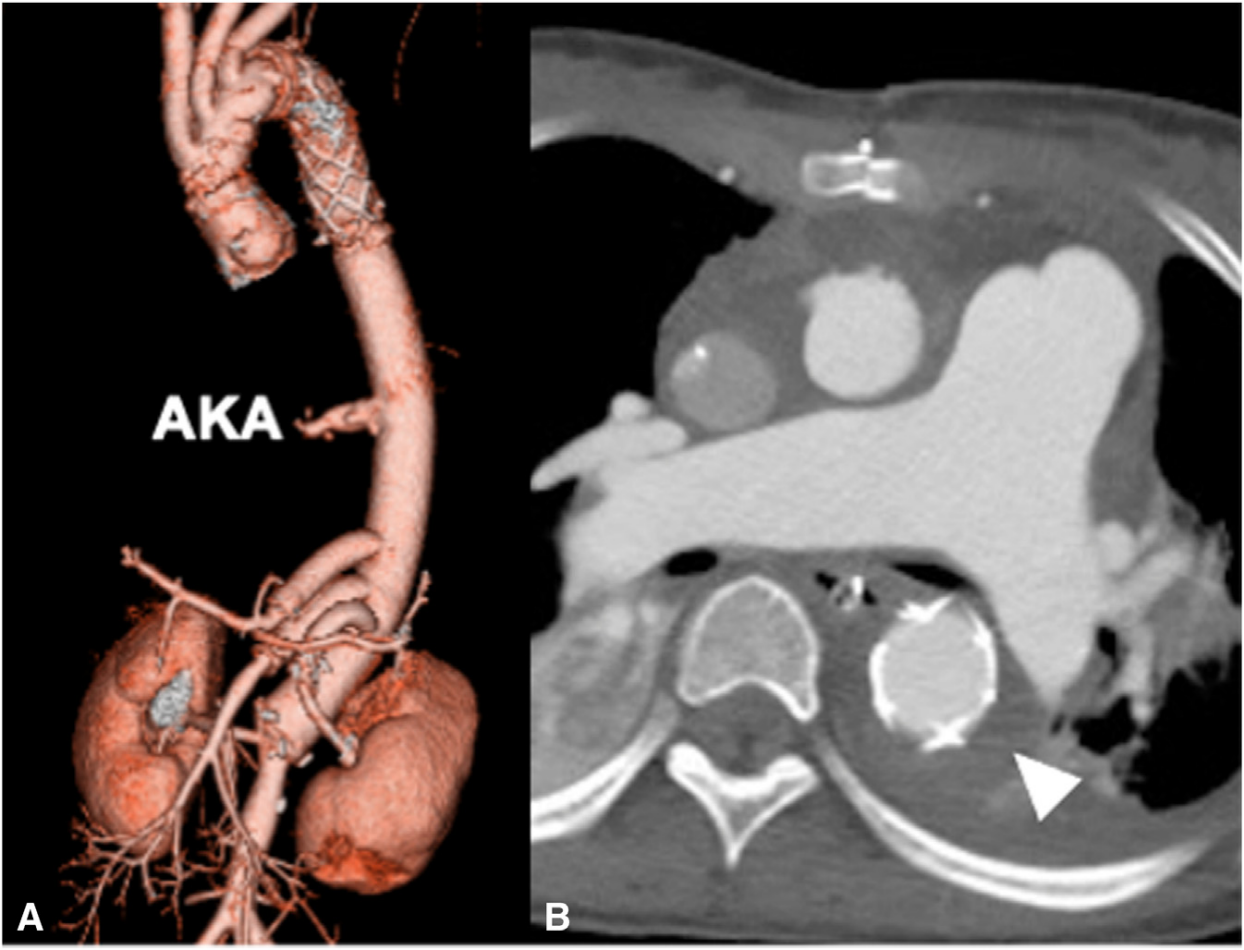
Postoperative computed tomography angiography. A, Successful staged extensive aortic replacement. Total arch replacement was performed using the frozen elephant trunk technique, which was anastomosed to a thoracoabdominal graft at the Th6 level. The Adamkiewicz artery (*AKA*) and reconstructed visceral branches were all open. B, False lumen around the frozen elephant trunk was thrombosed (*arrowhead*).
